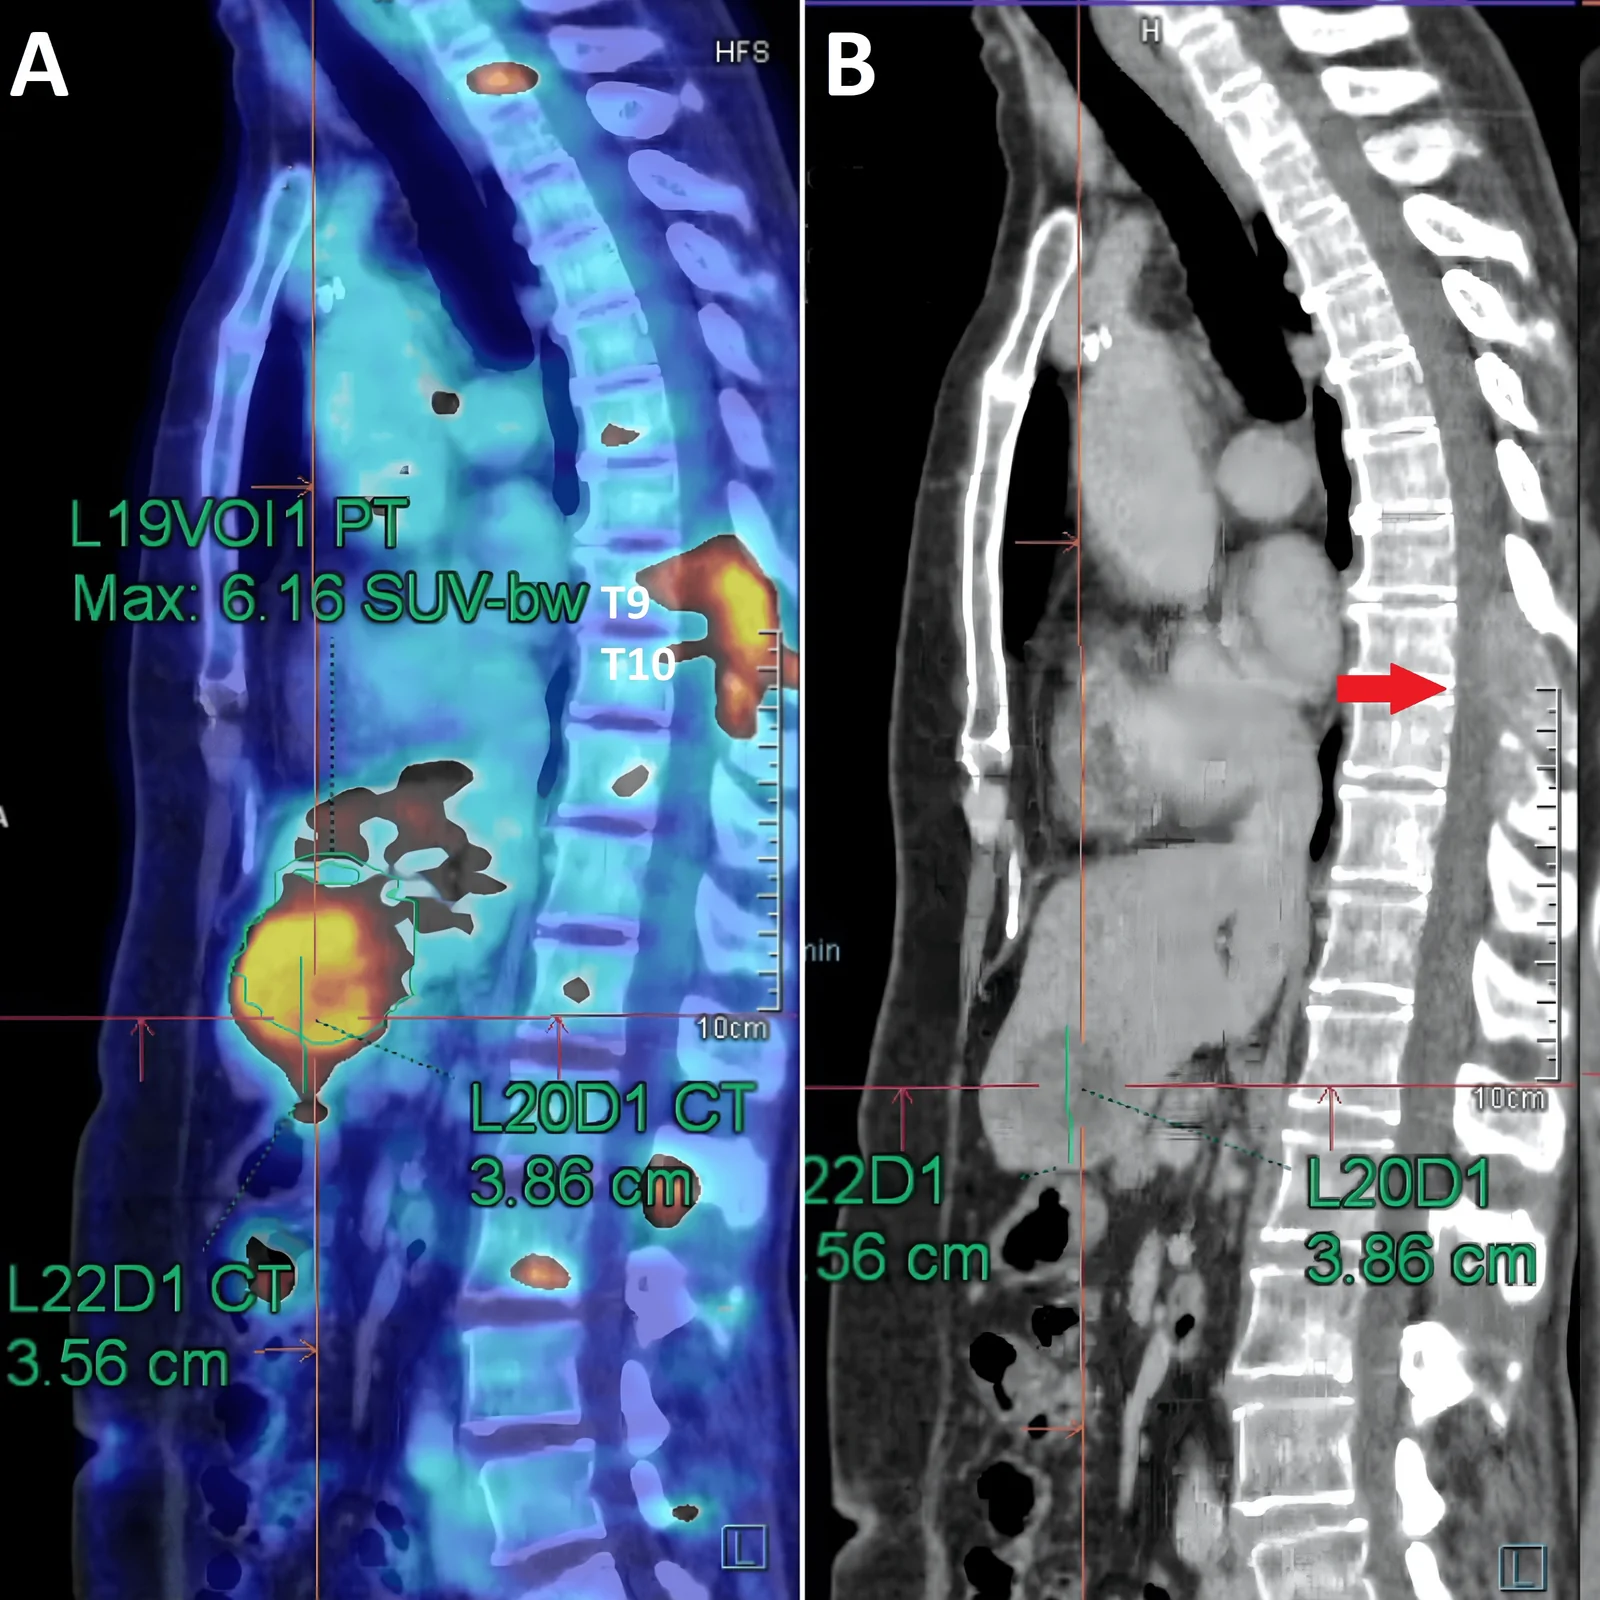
PET/CT images demonstrating left breast carcinoma with multiple bony metastases. (A) Coronal fused PET-CT image shows multiple hypermetabolic osseous lesions, including prominent metastases involving the posterior element of T9 and the spinous process of T10 with extension into the spinal canal (color overlay). (B) The corresponding coronal CT image highlights the destructive metastatic lesion at T10 (red arrow), with associated spinal canal narrowing and cord compression.
